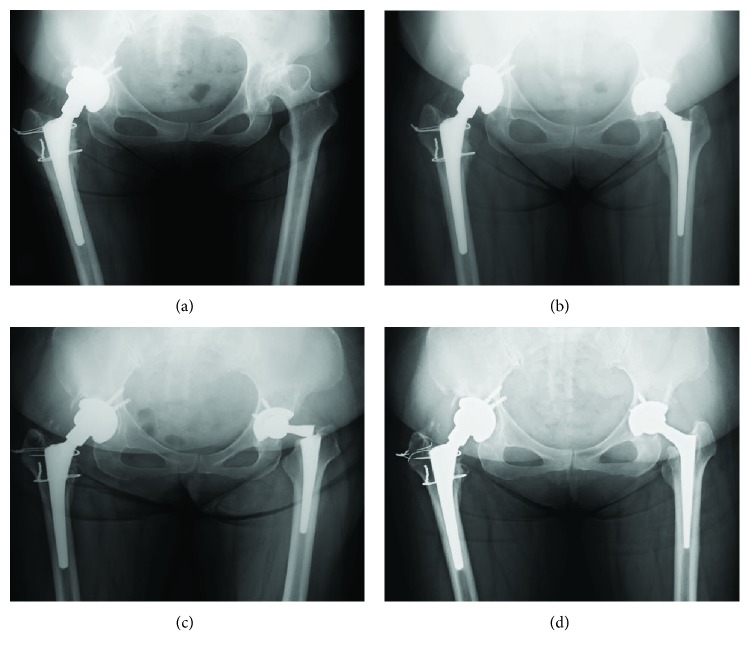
Radiographs. (a) Preoperative radiograph showing severe osteoarthritis of the left hip. (b) Postoperative radiograph of primary THA of the left hip using AHFIX Q stem (NAR #1). (c) Radiograph showing breakage of the AHFIX Q stem at the base of the neck. (d) Postoperative radiograph after revision THA of the left hip. THA: total hip arthroplasty.
